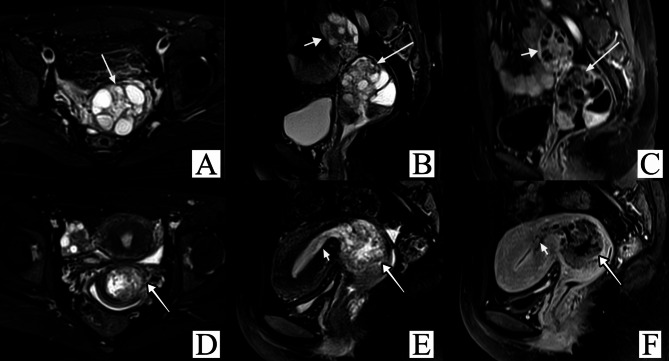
MRI of 2 PJS patients with G-EAC. A-C: A 31-year-old patient was classified as Grade 4. She was pathologically confirmed to have G-EAC concurrent with aLEGH and SCTAT with increased vaginal discharge for more than 10 years. Axial (A) and sagittal (B) T2WI showed dense microcysts surrounded by macrocysts (long arrow). Multiple small cysts could be observed in the left ovary (short arrow), and the cervical stromal ring was incomplete (B). The proportion of solid components was visible and less than 1/2. Sagittal contrast-enhanced T1WI (C) showed that the microcysts were moderately enhanced. D–F: A 31-year-old patient was classified as Grade 5. She was pathologically confirmed to have G-EAC concurrent with SCTAT. Axial (D) and sagittal (E) T2WI showed a diffuse and predominantly solid mass (long arrow) throughout the entire cervix. Local extension of lesions into the endometrium can be observed on sagittal T2WI (short arrow), and the cervical stromal ring was incomplete (E). Sagittal contrast-enhanced T1WI (F) showed that the proportion of solid components was more than 1/2 and was mildly enhanced.
